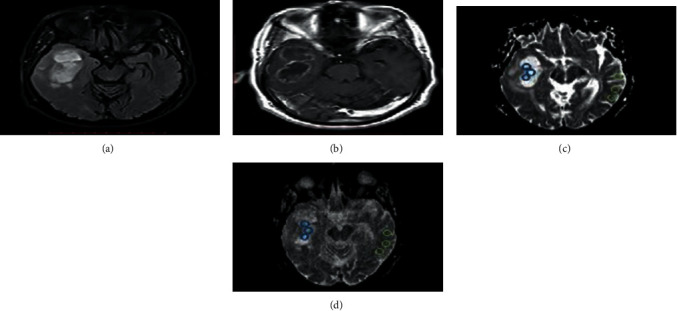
49-year-old man with GBM in the right temporal lobe. Based on the signal characteristics of T2WI and postcontrast T1WI, three ROIs were placed on the solid component of the tumor and three ROIs were placed on the contralateral NAWM on ADC images. Images (c, d). (a). On the T2 FLAIR sequence (TR/TE = 8800/120 ms), the GBM is hyperintense. (b). On T1WI SE (TR/TE = 410/9 ms) after contrast injection, the tumor is hypointense with ring enhancement. (c). ADC map at standard *b* value (TR/TE = 6100/152 ms; *b* = 1000s/mm^2^). (d). ADC map at high *b* value (TR/TE = 8600/152 ms; *b* = 3000s/mm^2^). The tumor is more hypointense on the ADC map obtained at a high *b* value than the ADC map obtained at standard *b* value.
